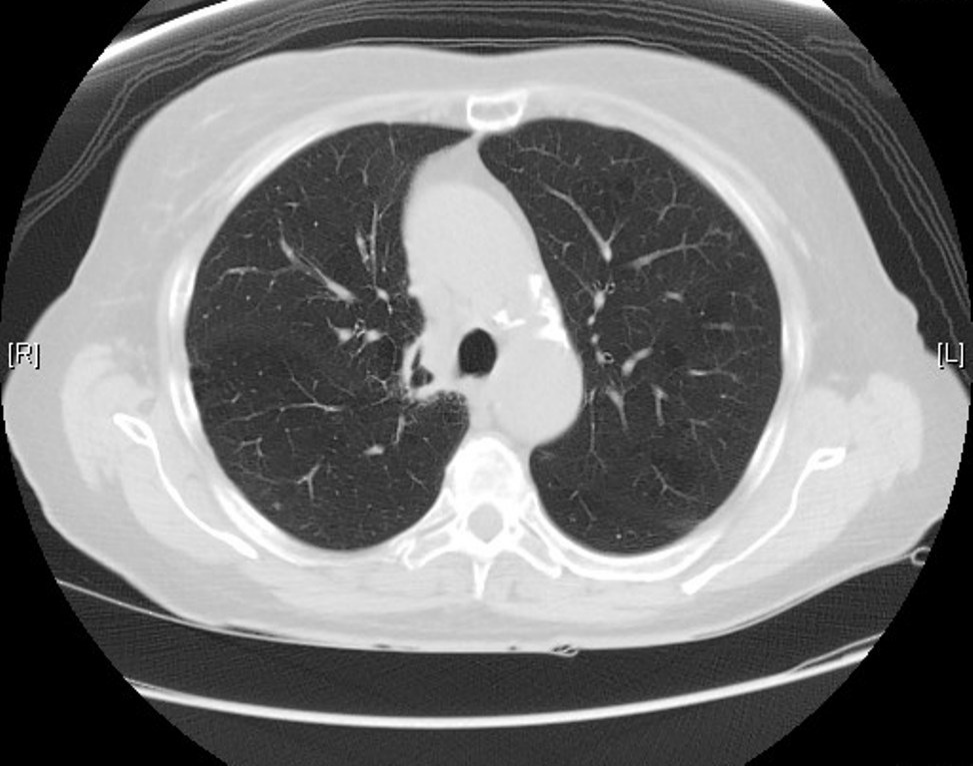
Post-chemotherapy CT scan of the chest from 2005 showing resolution of parenchymal nodular opacity
